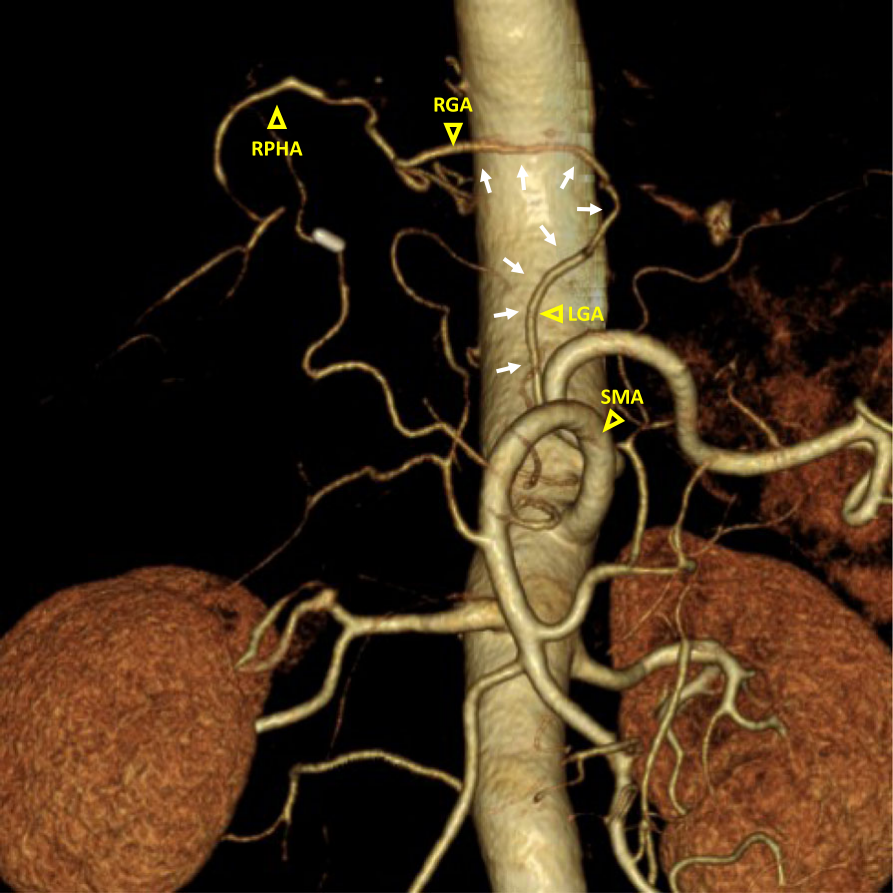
A postoperative three-dimensional CT angiogram. The gastric arterial arcade is shown (white arrows)
